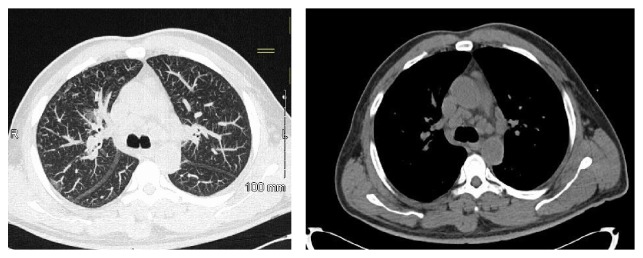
CT chest without IV contrast. Computed tomography without intravenous contrast showing mediastinal and hilar adenopathy.
